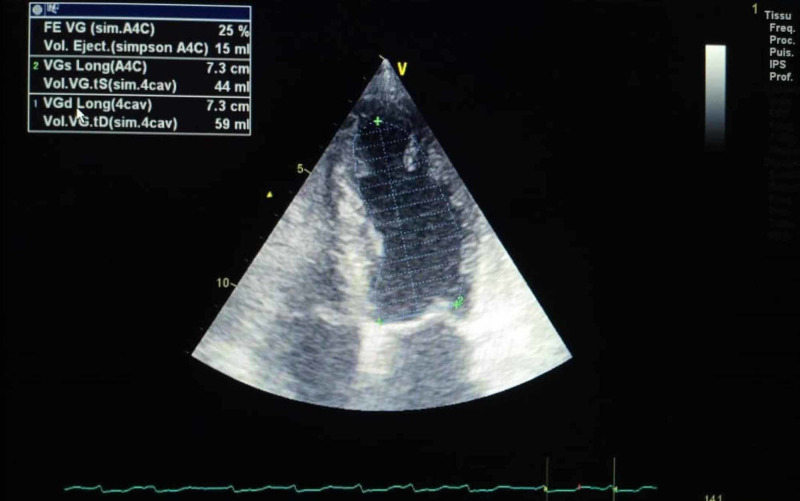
Transthoracic echocardiography showing severe LV systolic dysfunction EF 25% LV: left ventricle; EF: ejection fraction
